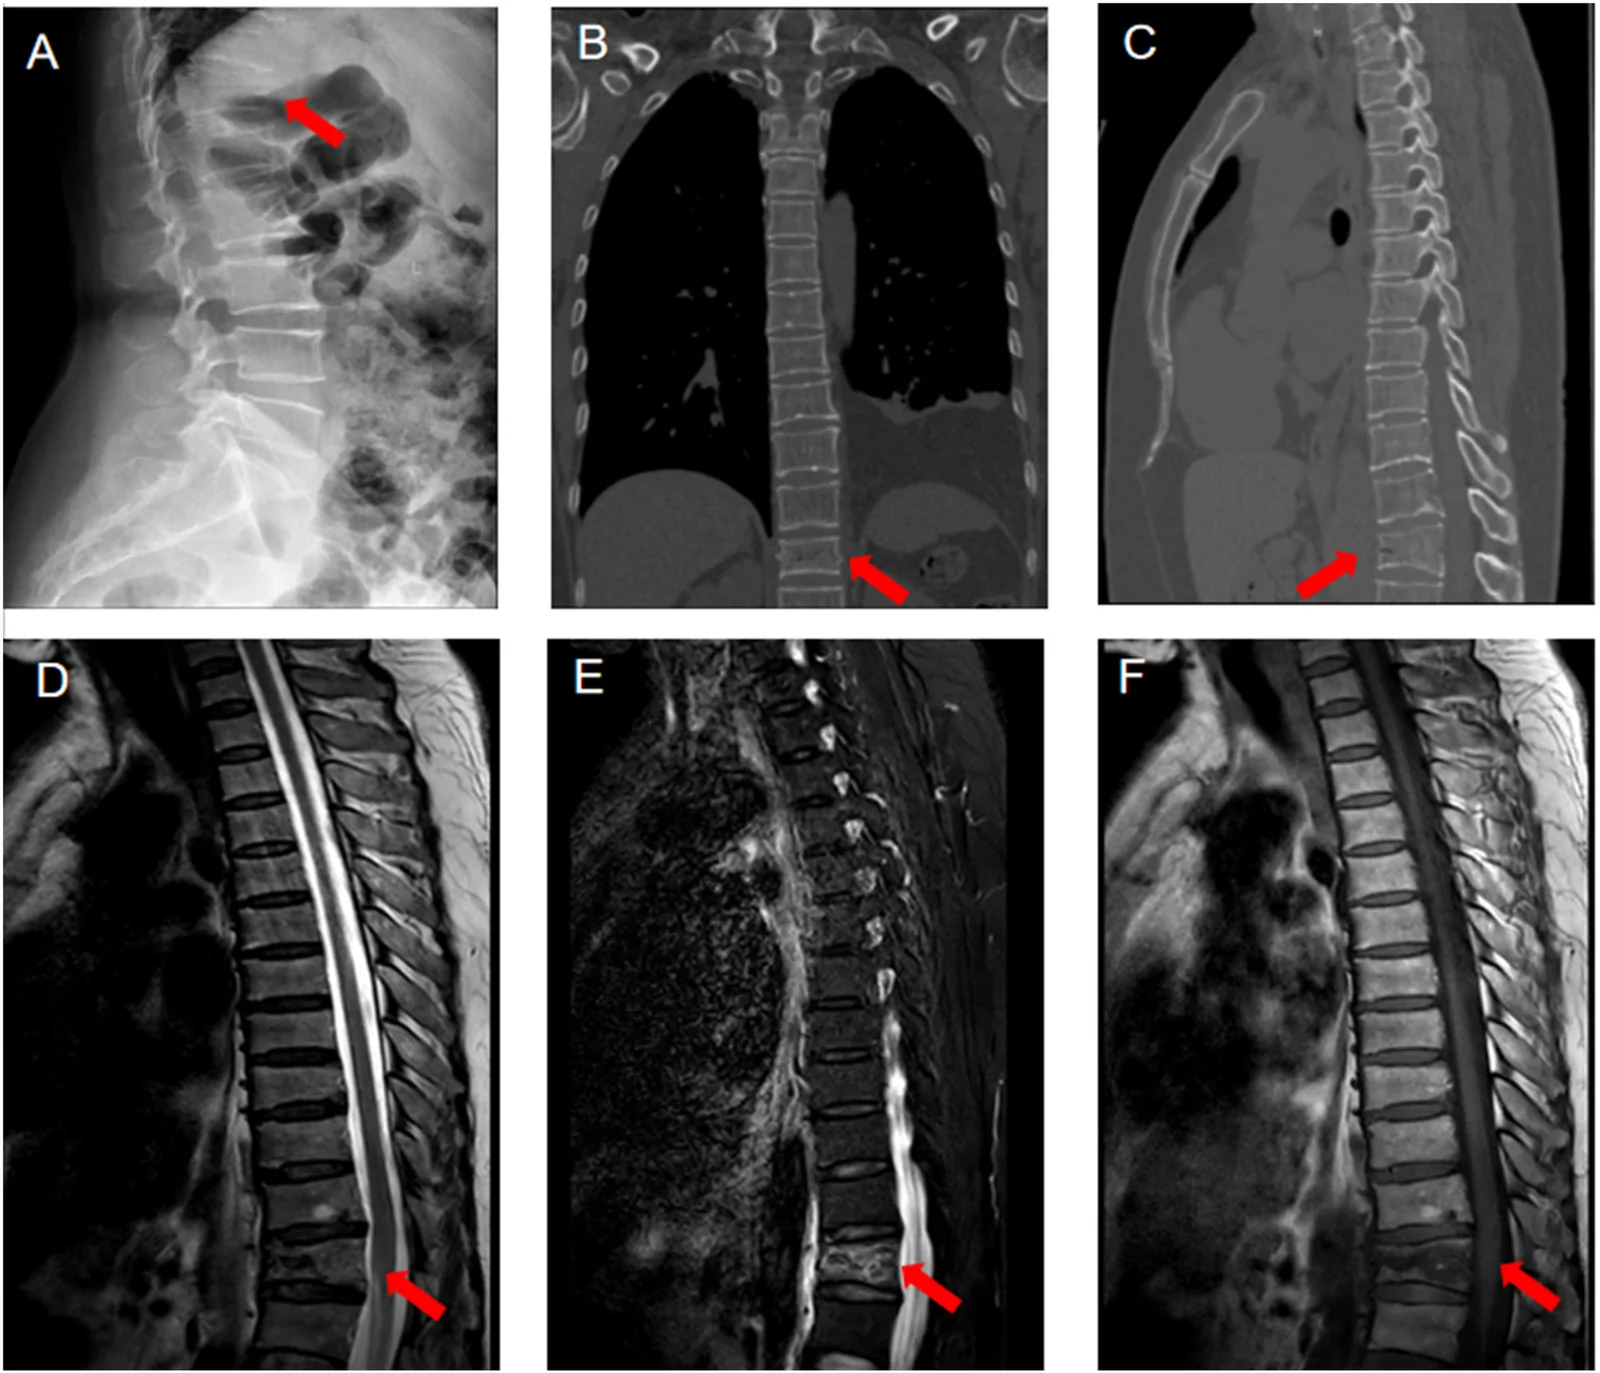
Plain radiography, CT, and MRI images (A–F) demonstrating T12 vertebral compression fracture with acute bone marrow edema.
